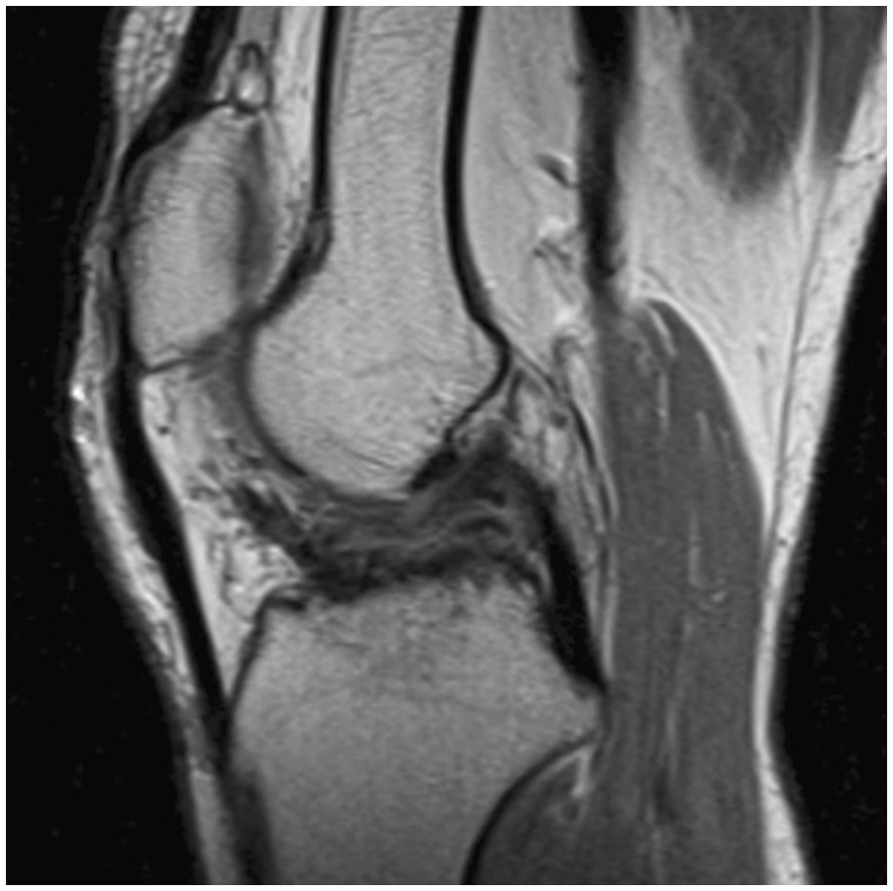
MRI findings. Sagittal fast-spin-echo magnetic resonance imaging shows the disappearance of the distal ACL attachment.
